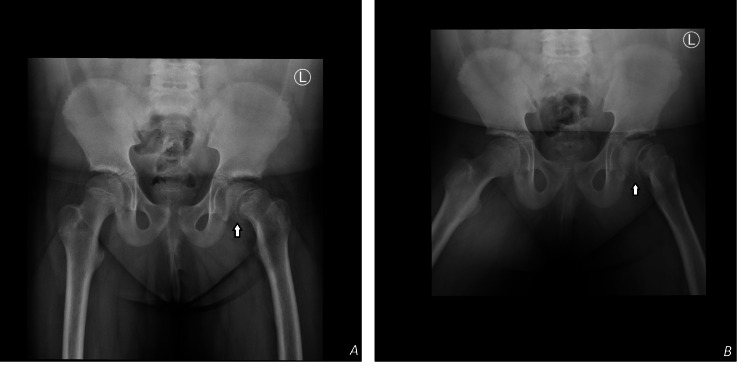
X-rays of the pelvis, AP (A) and frog-leg (B) views, showing SUFE of the left hip joint (white arrows). SUFE: Slipped Upper Femoral Epiphysis; AP: Anteroposterior
